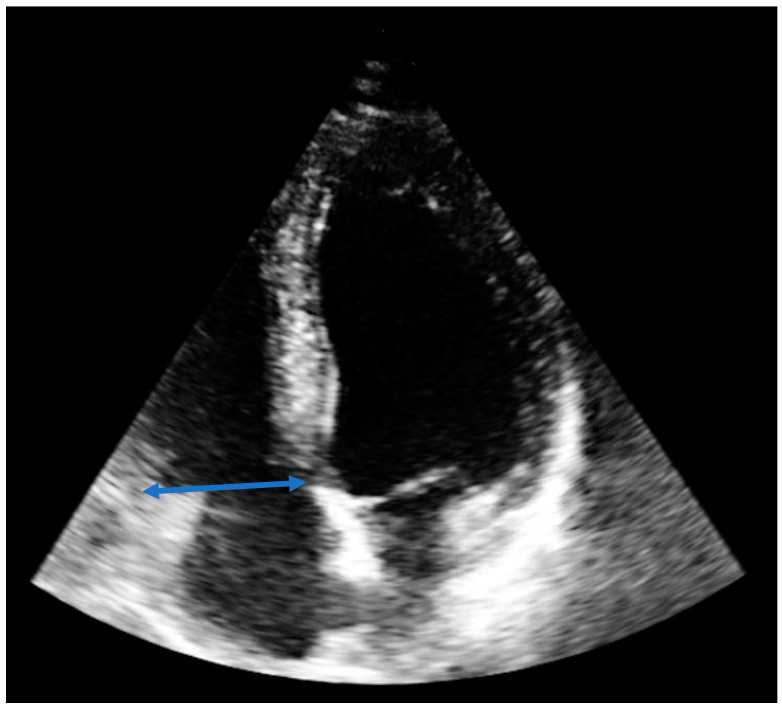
Transthoracic echocardiogram before discharge showing a normalized right ventricle with a right ventricular end-diastolic diameter (RVEDD) of 3.7 cm (arrow).
